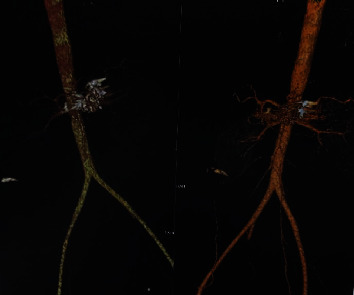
Control CT scan after radiological embolization of the aneurysm (3D reconstruction). CT, computer tomography.
